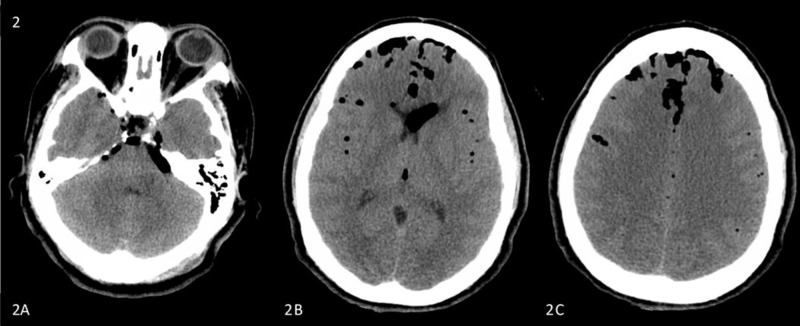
Picture demonstrated the progression of the pneumocephalus (PNC). Repeat axial computed tomography of the brain obtained a few days later: (A) Increased air in the posterior fossa causing mild mass effect on the pons. (B) and (C) Slightly increased PNC in the frontal and parafalcine regions.
